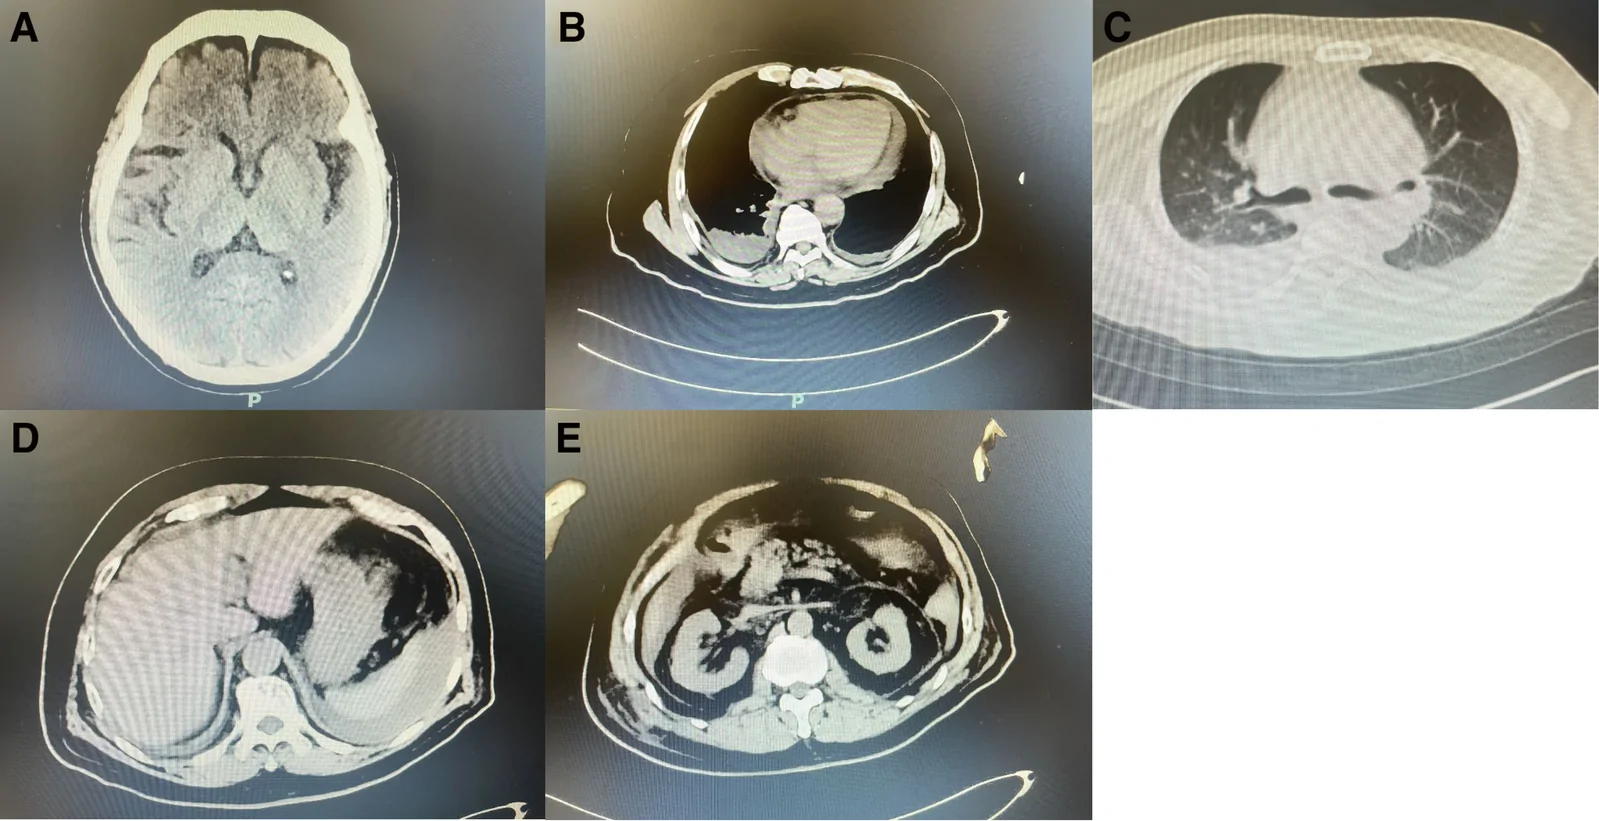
Representative CT scans. (A) Brain CT showing no acute intracranial abnormalities; (B and C) chest CT revealing small bilateral pleural effusions and no focal consolidation; (D and E) abdominopelvic CT showing no significant ascites or organ abnormalities. CT = computed tomography.
